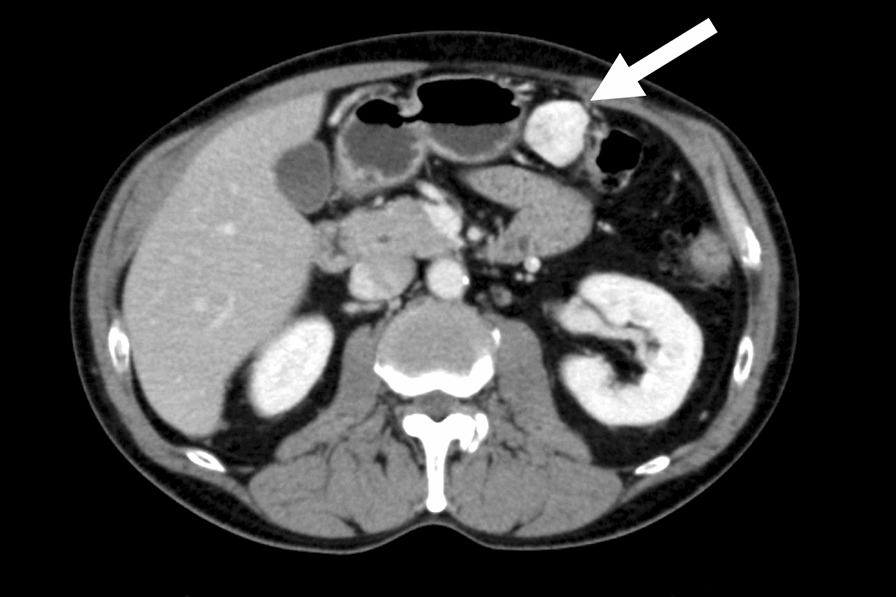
Representative contrast-enhanced computed tomography images in this case. A contrast-enhanced computed tomography scan showing an abdominal mass approximately 3 cm in size in the greater omentum (arrow indicates tumor)
